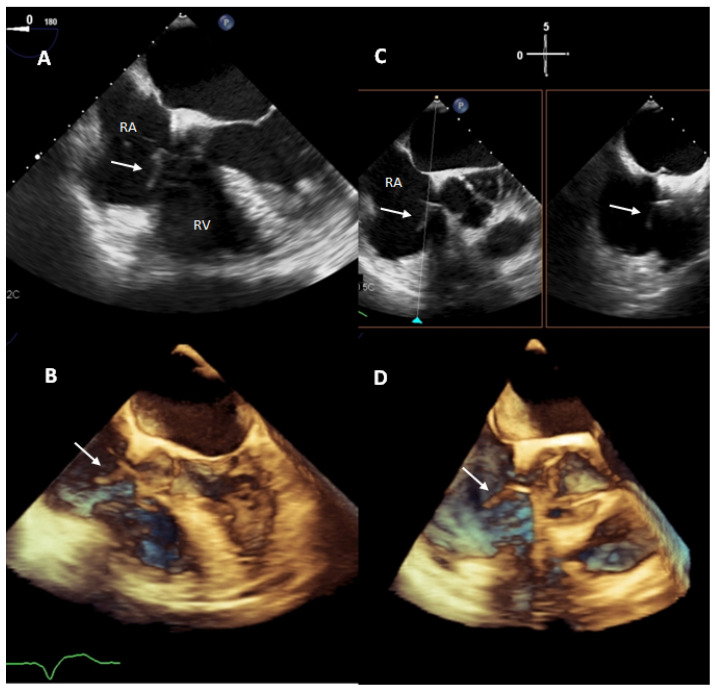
Transesophageal echocardiogram, 5 chamber view, showing a vegetation (19 × 6 mm) adherent to pacemaker’s ventricular lead in 2D (panel (A), white arrow) and 3D (panel (B)). The same vegetation is also shown in right ventricular inflow-outflow view (and its orthogonal plane-bicaval view) in 2D (panel (C)) and in 3D (panel (D)). RV: right ventricle; RA: right atrium.
